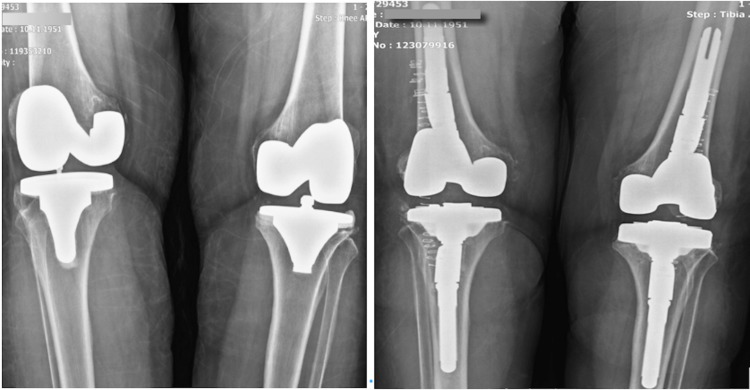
Case 1 Preoperative and Postoperative Knee X-Ray
